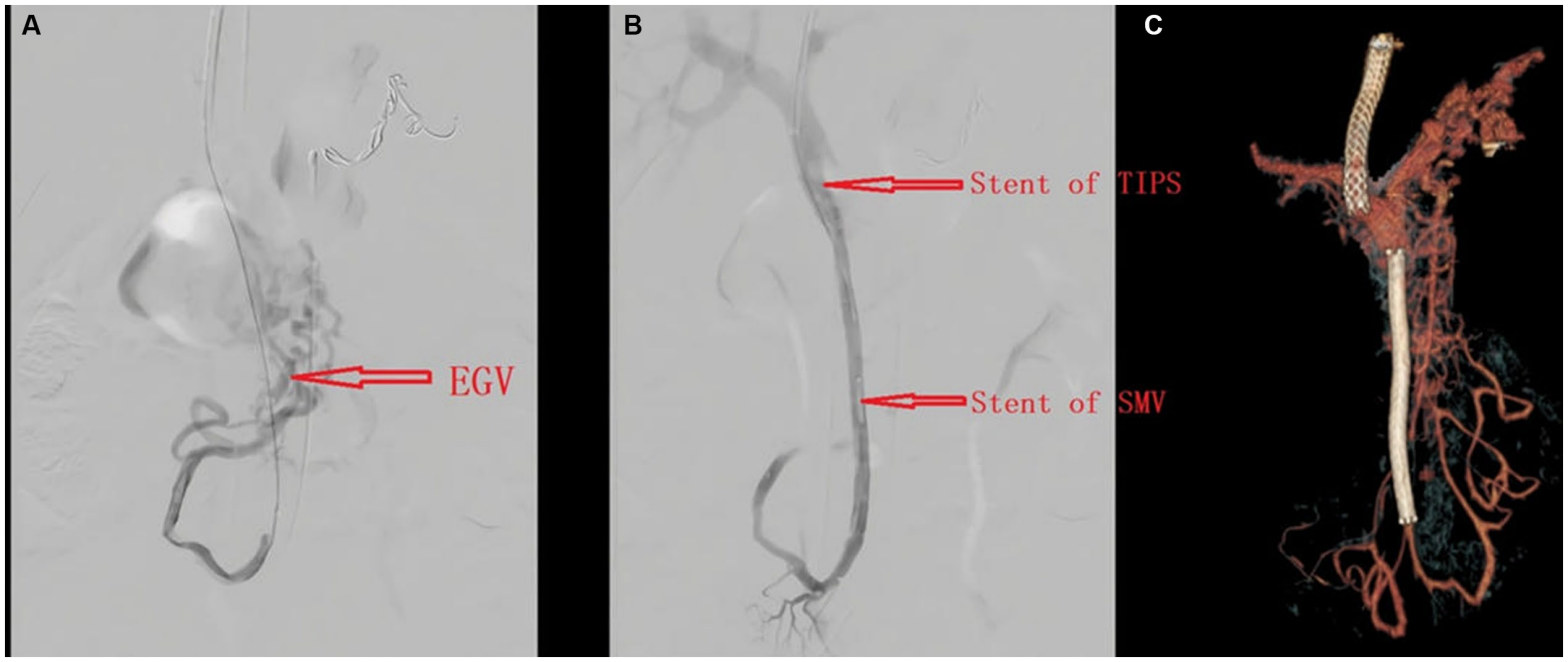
Angiography before (A) and after (B) TIPS placement; CT angiography after TIPS (C).
